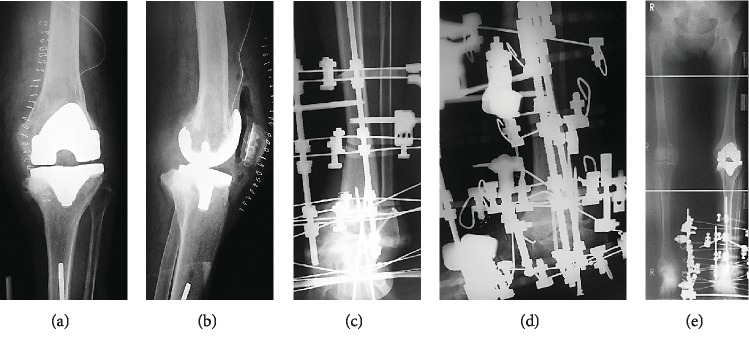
Postoperative radiographs of the knee and ankle. (a, b) A 5 mm defect is observed at the medial tibia. After drilling, cement is used as filling. The posterior-stabilized (PS) type is fixed with cement. (c–e) Ilizarov external fixation is performed on the ankle joint. The bone is repositioned to a neutral position without shortening.
